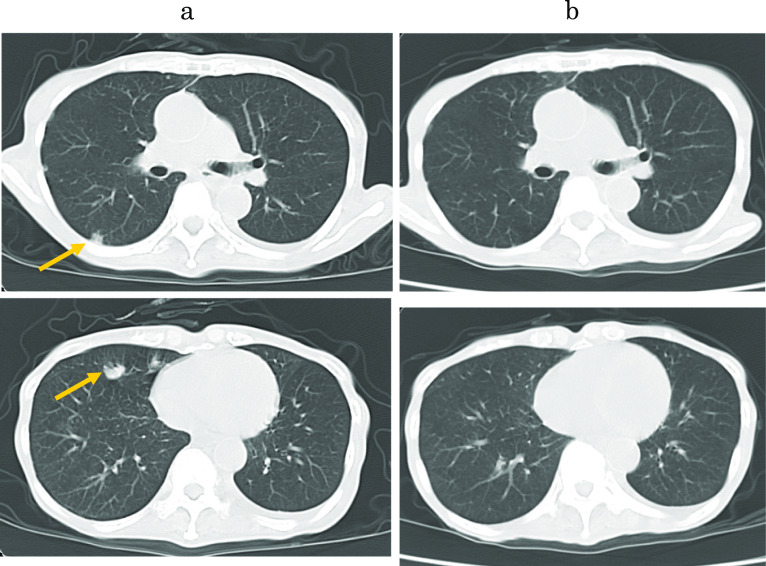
Thoracic CT imaging. a CT scan showed multiple lung metastases in the right lung (yellow arrow). b The lung metastases disappeared after S-1 plus trastuzumab chemotherapy
